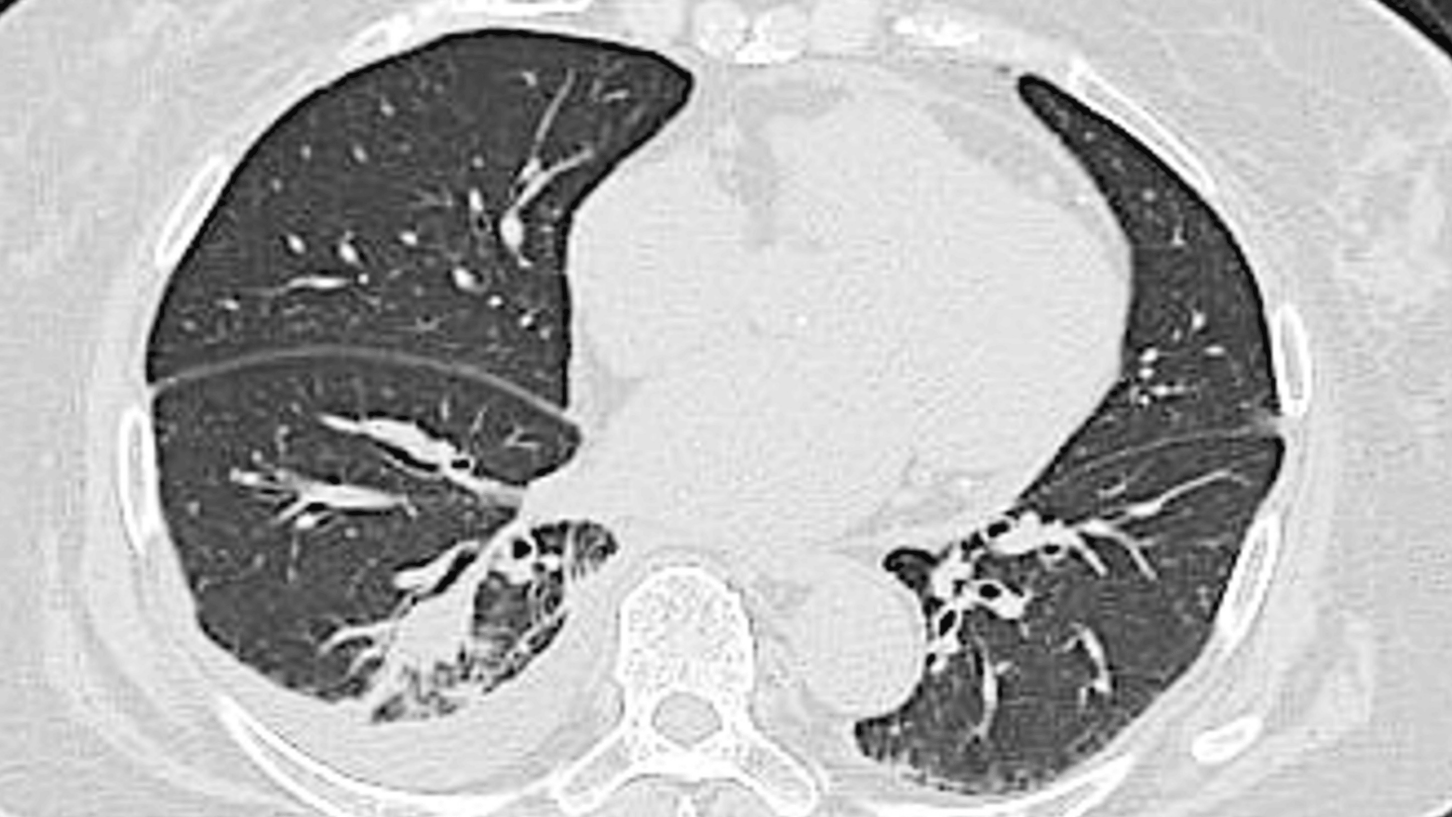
Chest computed tomography scan one month after selpercatinib administration shows a reduction in tumor size.
